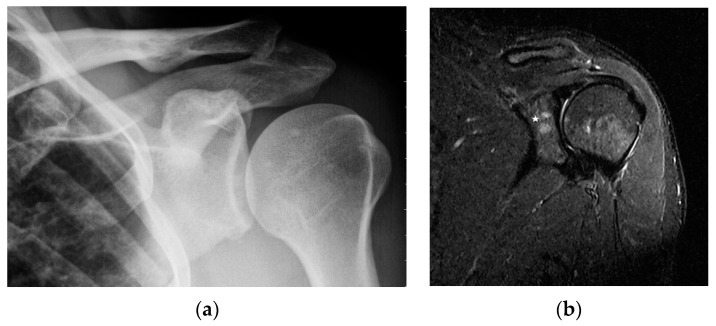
Radiograph (a) and magnetic resonance of the shoulder joint. T1-weighted image with fat saturation and with contrast administration (b). 40-year-old patient with sarcoidosis. Two contrast-enhanced lesions in the acetabulum of the joint ((b), asterisk) not visible on X-ray (a).
